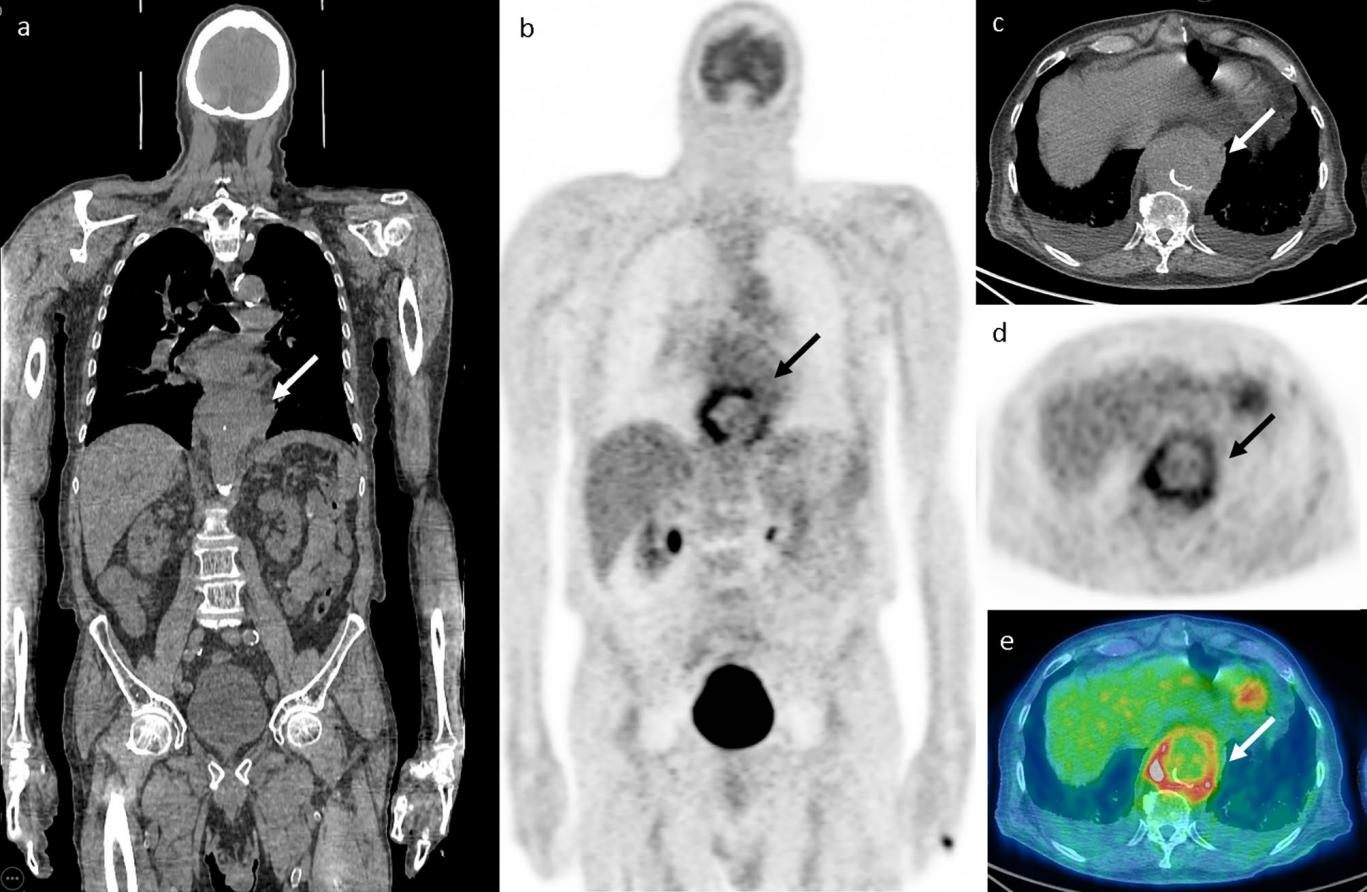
Infectious focus in an aortic pseudoaneurysm. A 73-year-old male presented with dyspnea and was diagnosed with *Staphylococcus aureus* bacteremia and empyema that was drained. FDG PET/CT images (A, B coronal slices, C, D, E axial slices at the level of the lower chest) show pathological FDG uptake in a thoracic aortic pseudoaneurysm (arrows). The patient underwent endovascular aortic stent insertion and was treated with intravenous antibiotics followed by chronic suppressive oral treatment. In a follow-up visit 6 years later, the patient has no evidence of disease.
